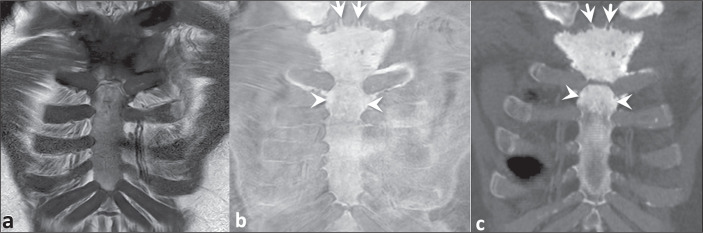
A 56-year-old man with SAPHO syndrome. A T1-weighted MRI sequence (a)
